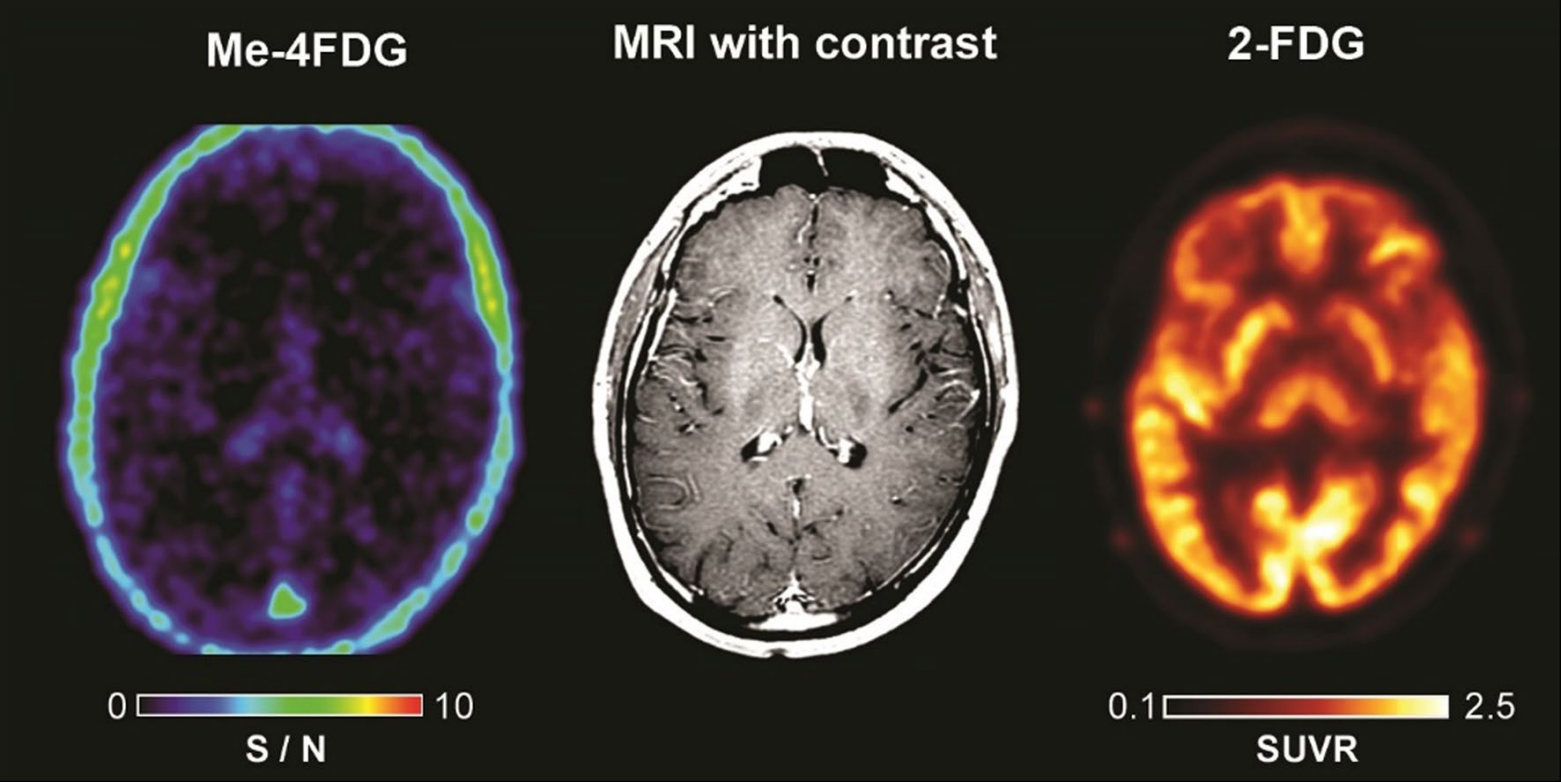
Me-4FDG, MRI, and 2-FDG PET scans on 26-year-old control subject. The Me-4FDG S/N (SUVR/BG) scale 0–10 is shown for the NIH color scale, and the 2-FDG SUVR scale is 0.1–2.5 for the Hot-Iron color scale
